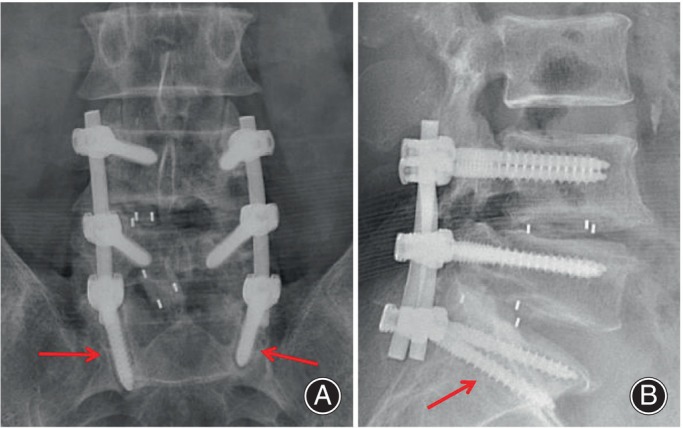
Pedicle screw loosening on anteroposterior (A) and lateral (B) radiographs. The radiolucent line indicated by red arrow.
